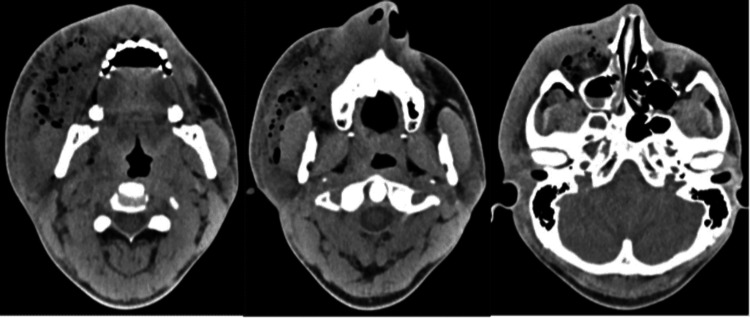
Facial CT on post injury day two with extensive subcutaneous emphysema on R face extending from the mandible to the calvarium with involvement of the inferior orbit
